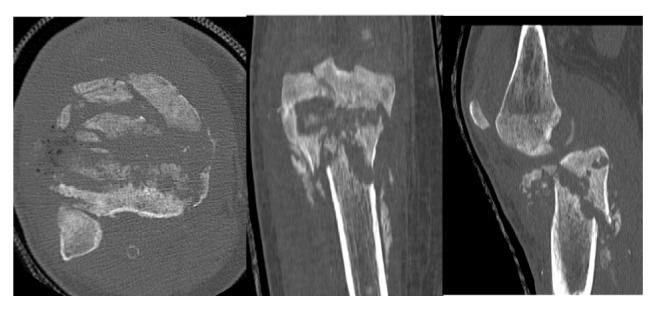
CT-scan after plate removal of the right knee.
